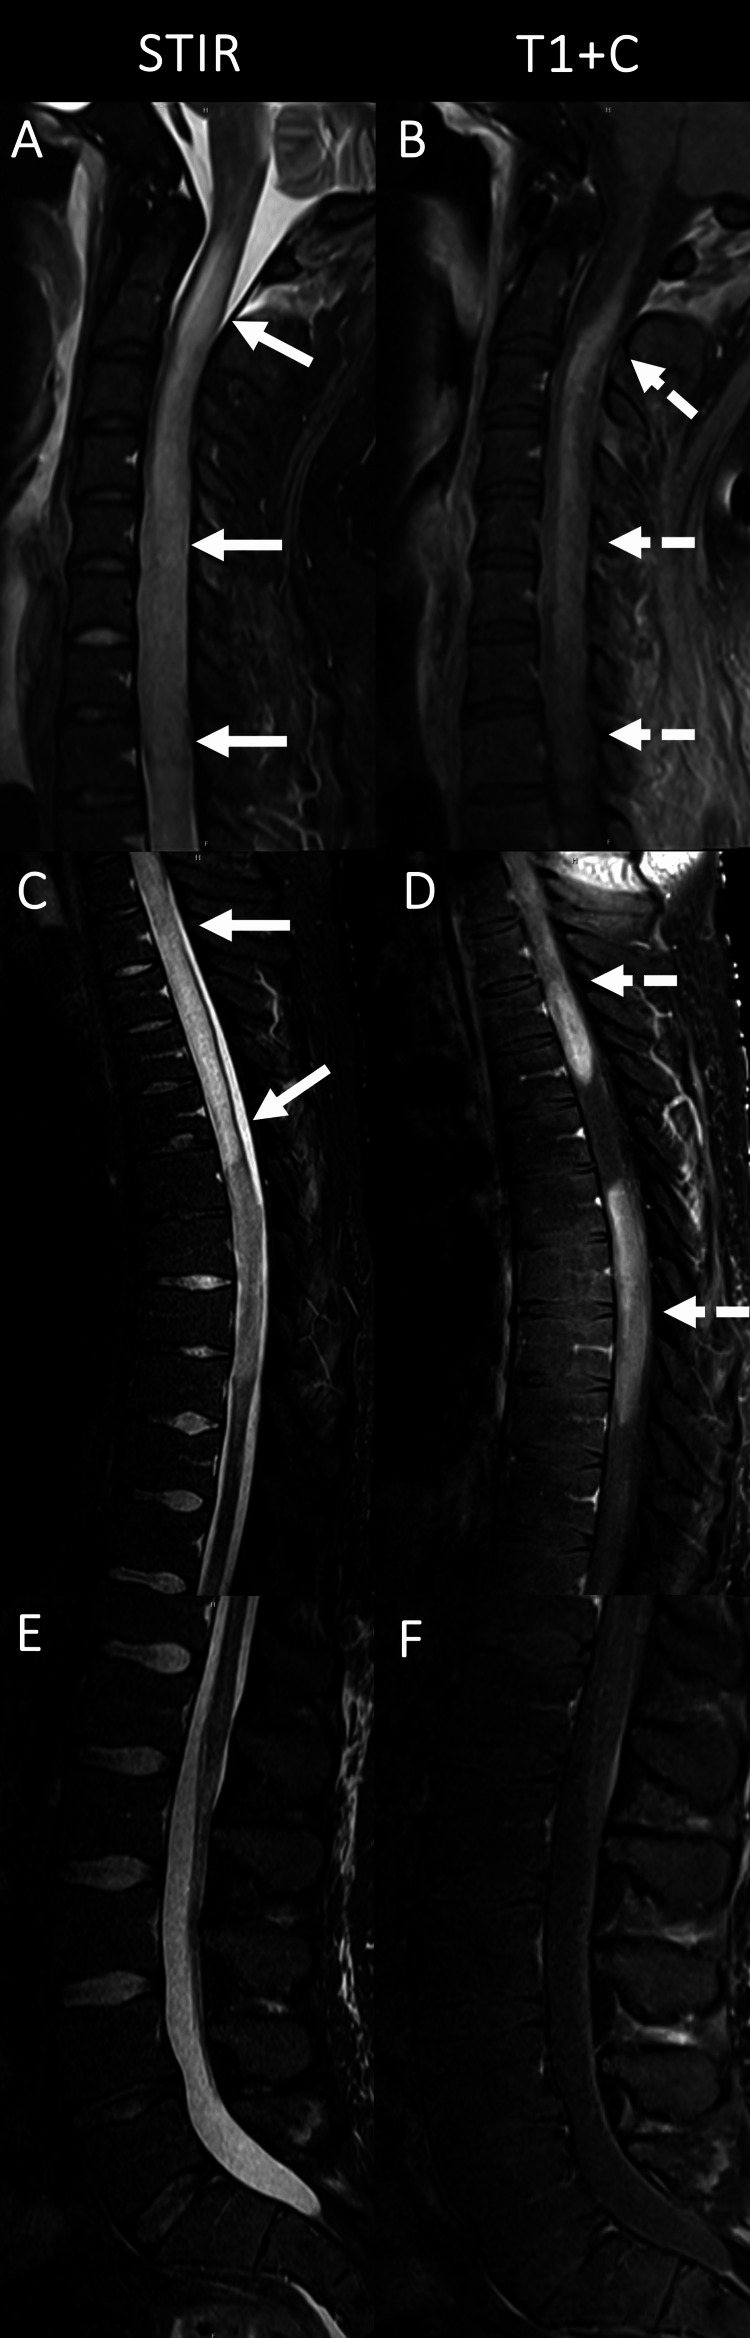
Sagittal MRI images of the spine obtained 38 months after diagnosis, four months after the MRI in Figure 3, showing marked progression of gliomatosis throughout the spinal cord Solid arrows in A and C show diffusely infiltrating expansile STIR hyperintense spinal cord lesions spanning the C1-T10 levels. B demonstrates ill-defined diffuse enhancement throughout the cervical cord (dashed arrows) while D demonstrates several more focal regions of the long segment and masslike enhancement within the thoracic cord (dashed arrows). The lumbar spine remains unremarkable. STIR: short tau inversion recovery (STIR)
